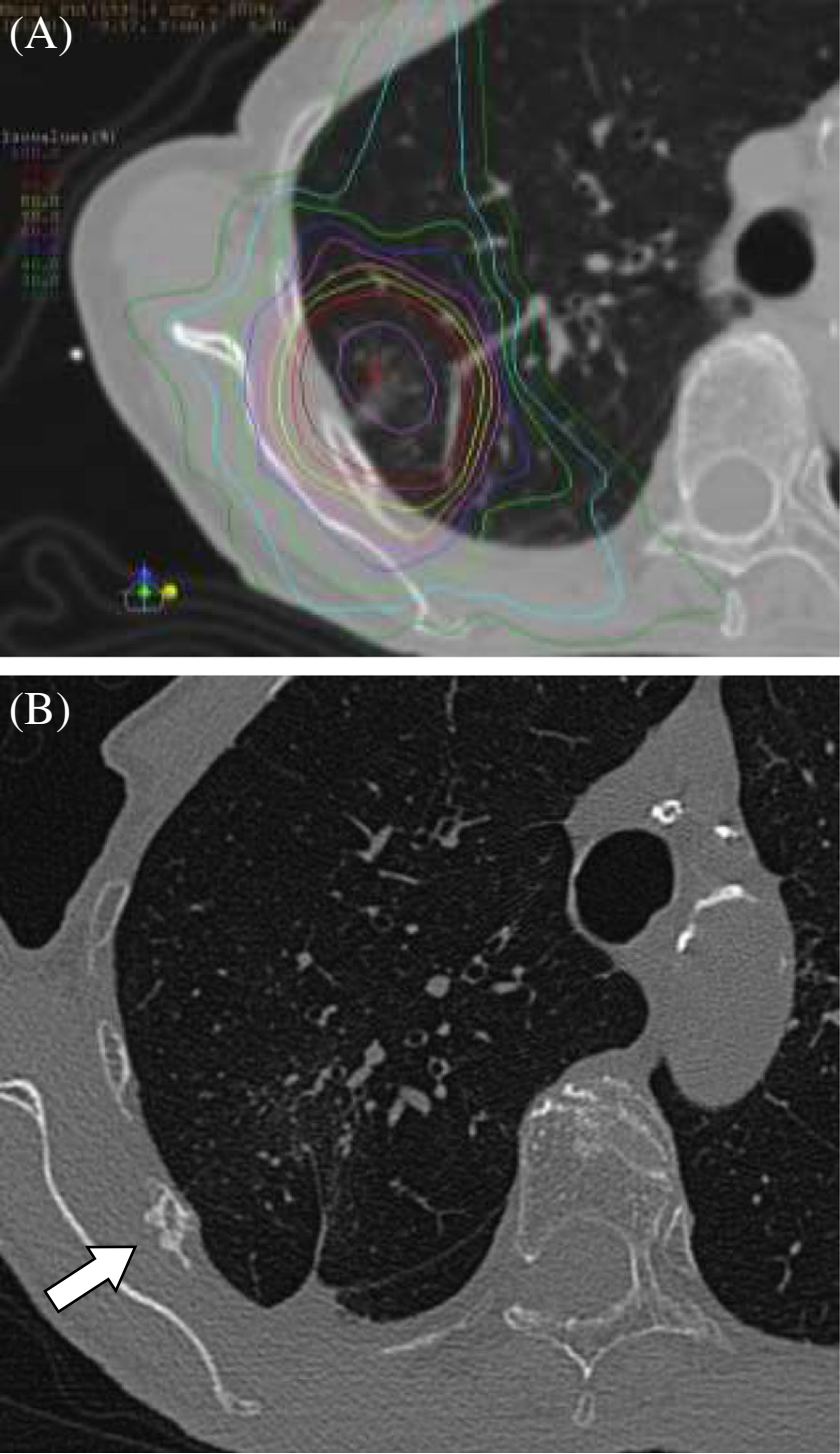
An 83-year-old woman with adenocarcinoma after SBRT with a total dose of 56 Gy given in 7 fractions. (A): Dosimetry overlaying CT with bone window shows the 95% iso-dose line on the right 5th rib. (B): Rib fracture was noted at 36 months after SBRT (arrow).
